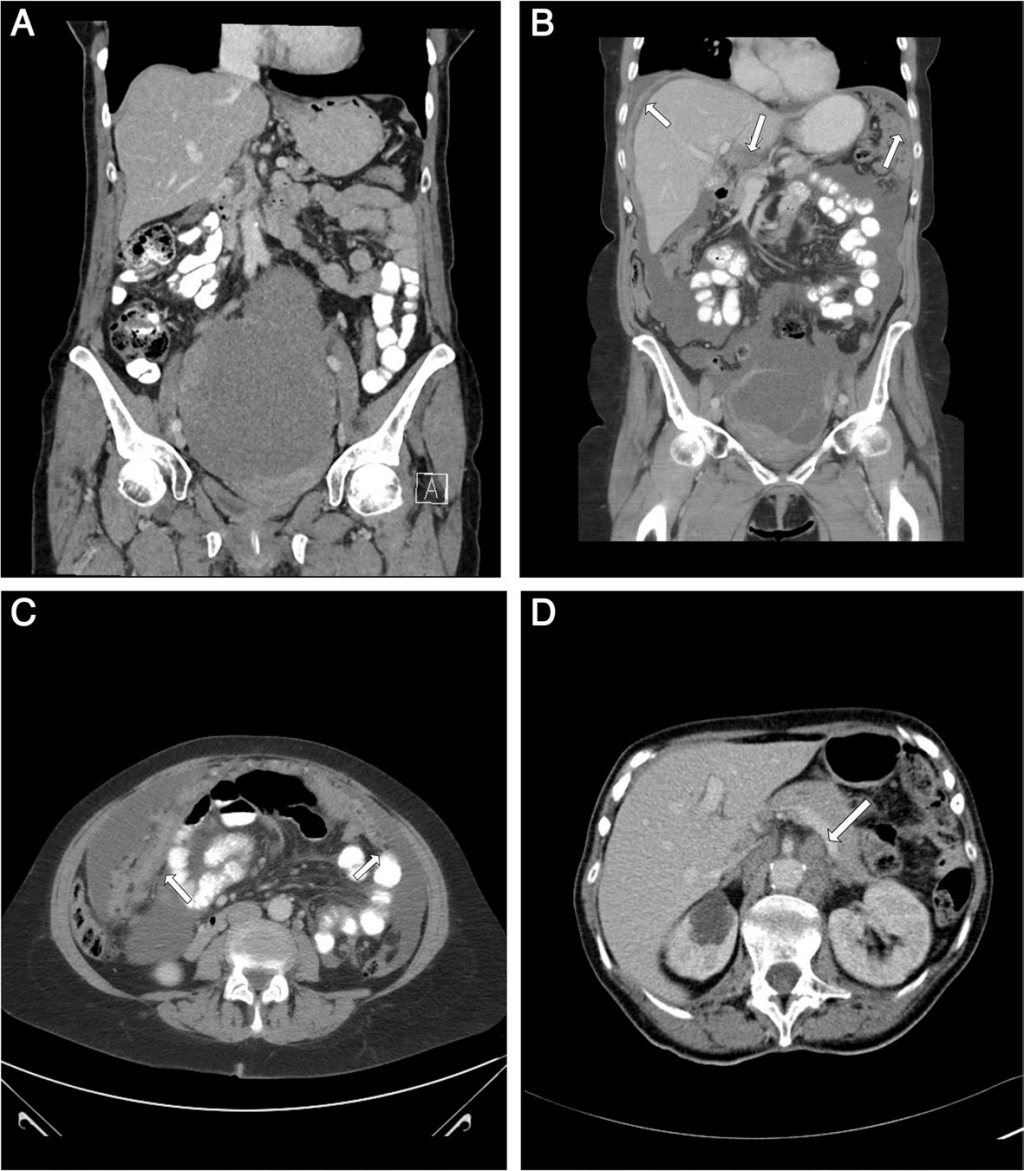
A A coronal abdominal CT showing a left sided ovarian tumor mass, however no signs of additional spread in the abdomen (0 points). B (coronal) and C (axial), same patient (6 points): An abdominal CT showing involvement of right and left diaphragm (2 points), liver hilum (1point), small intestines (1 point, image C), coeliac trunc (1point) (not shown in image), and cardiophrenic lymph nodes (not shown in image) (1 point). D An axial abdominal CT showing involvement of coeliac trunc and lymph nodes above the renal artery level, a short score of 2 points
